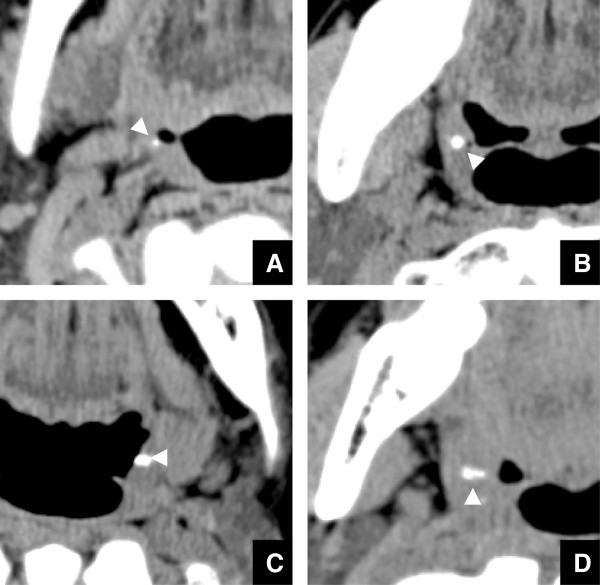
Four kinds of tonsillolith shapes on CT images. A) A dot shape of tonsilloliths (arrowhead) is shown on axial CT images. B) A round shape of tonsilloliths (arrowhead) is shown on axial CT images. C) An oval shape of tonsilloliths (arrowhead) is shown on axial CT images. D) An irregular shape of tonsilloliths (arrowhead) is shown on axial CT images.
